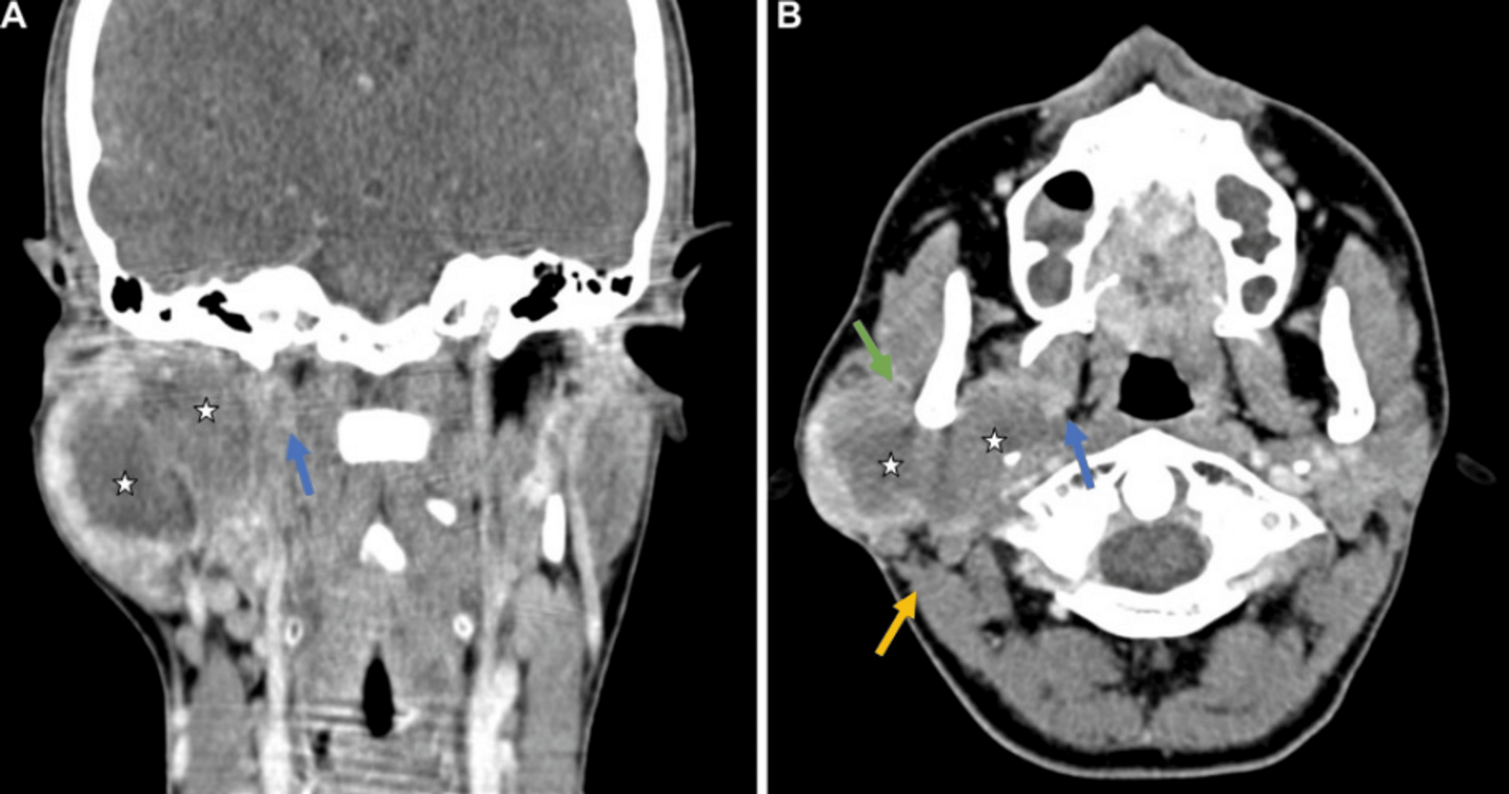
Contrast-enhanced computed tomography of the neck (A) Coronal view. (B) Axial view. A 5.6 cm solid, irregular lesion in the superficial and deep lobes of the right parotid gland, demonstrating peripheral enhancement and central areas of necrosis (stars). Infiltration of the parapharyngeal space and the medial pterygoid muscle is observed (blue arrows). The lesion contacts the posterior margins of the masseter (green arrow) and sternocleidomastoid (yellow arrow) muscles.
